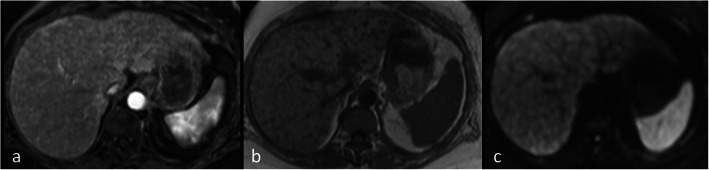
Hypervascular liver metastases on MRI. Liver MRI in a 52-year-old woman with breast cancer demonstrates multiple slightly T1 hyperintense lesions in the liver on the T1-W image (a) which show increased enhancement in portovenous phase (b) and impeded diffusion on b750 DWI (c)
